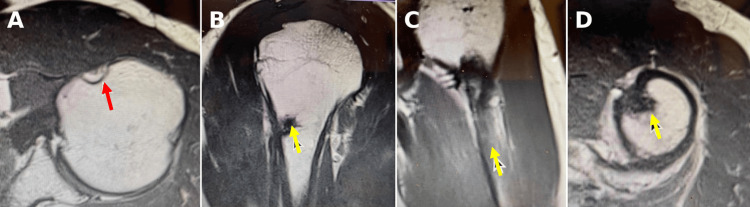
Twelve-month postoperative T1-weighted MRI of the left shoulder. (A) Absent LHBT in the bicipital groove (red arrow). (B-D) Subpectoral biceps tendon in the intraosseous tunnel (yellow arrows). MRI: magnetic resonance imaging, LHBT: long head biceps tendon.
